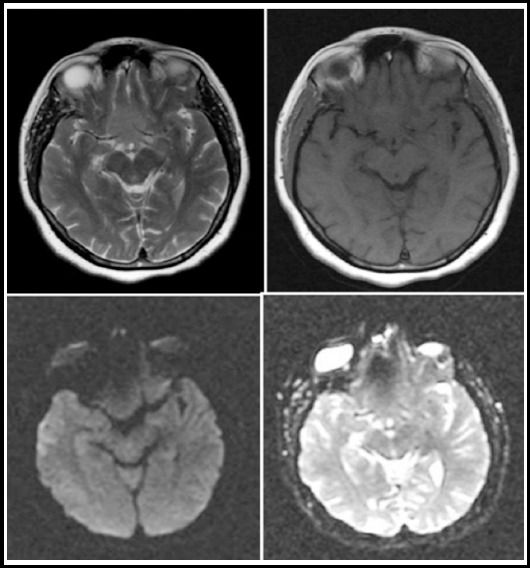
Axial T2WI, T1WI, DWI and ADC images show a benign suprasellar meningioma without diffusion restriction on DWI/ADC.
